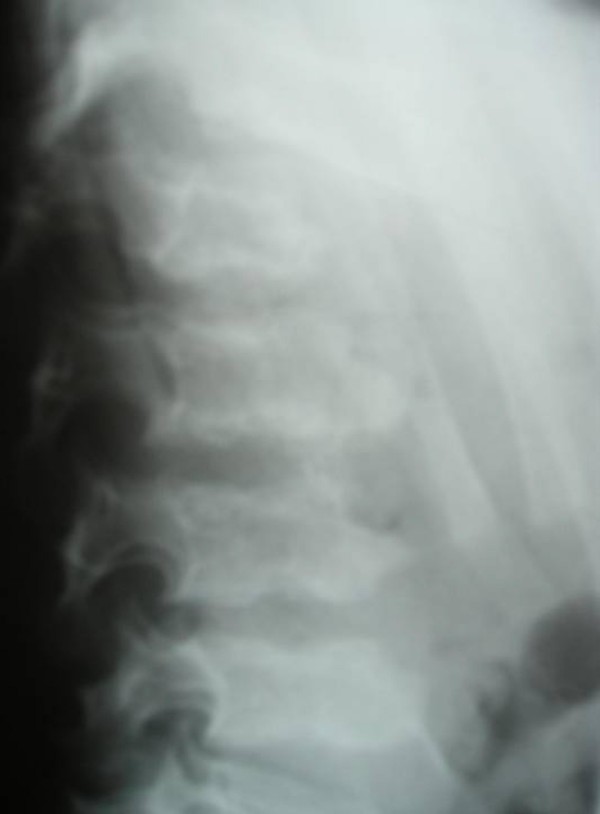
Lateral spine radiograph showed significant platyspondyly. A central tongue/anterior beaking associated with narrow discs was evident.
